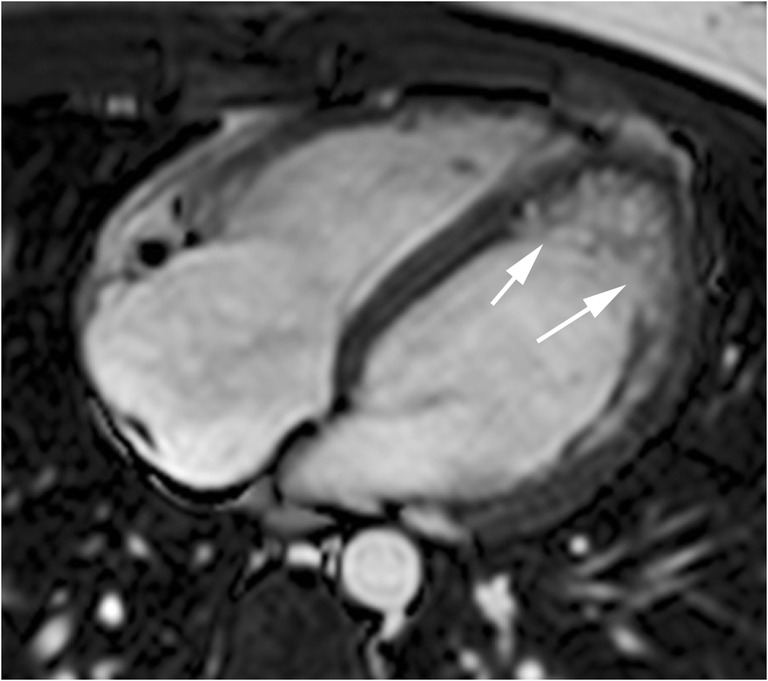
22-year-old man with a family history of HCM, genotype positive, phenotype negative. End-diastolic SSFP cine MR images showing prominent trabeculations in the LV apex (arrows) and normal miocardial thickness. These MRI features are indistinguishable from LV non-compaction
